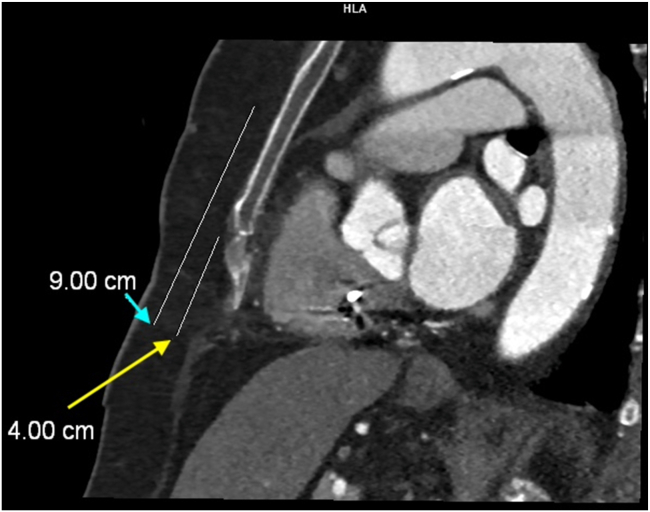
Left lateral view of chest computed tomography. *Yellow arrow:* Distance between the xiphoid process to the xiphisternal junction, 4 cm. Ring 2 of the Epsila extravascular lead was planned to position at 1–2 cm caudal to the xiphisternal junction. *Blue arrow:* Distance between the xiphoid process to the level of the tracheal carina, 9 cm.
